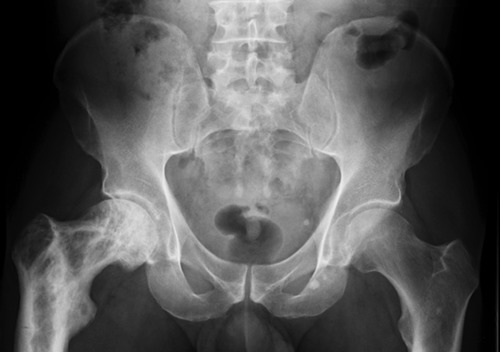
X‐ray features of PDB. Pelvic radiograph from a patient with PDB affecting the upper right femur showing alternating areas of osteolysis and osteosclerosis in the greater and lesser trochanters and femoral neck; loss of distinction between the cortex and medulla in the upper femur; bone expansion and deformity of the affected femur; and a pseudofracture on the lateral aspect of the femur opposite the lesser trochanter.
